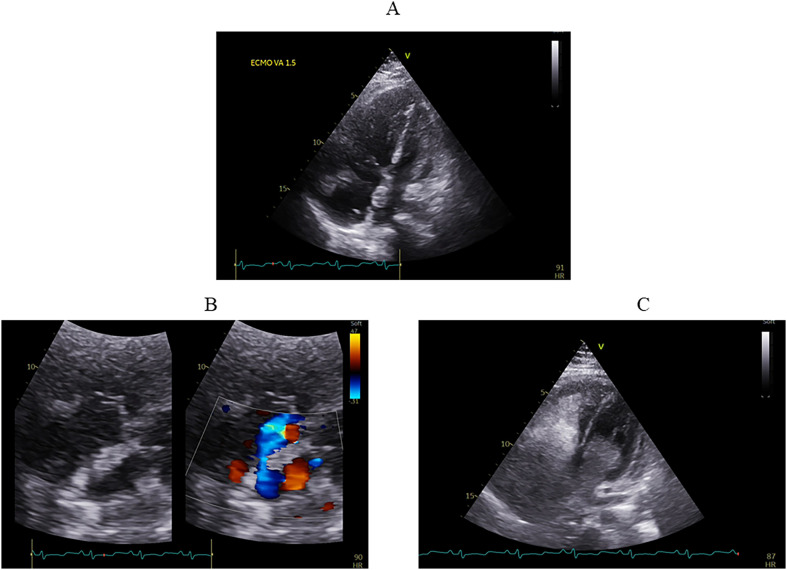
A. Apical four-chamber view on transthoracic echocardiography performed before atrioseptostomy (veno-arterial ECMO flow = 1.5 L/min). The view showed a dilated right ventricle, a compressed D-shape left ventricle, and a flattened septum. B. Transthoracic echocardiography with Color Doppler. A turbulent blood flow is visible through the interauricular septum. The flow appears bidirectional (with both blue and red signals mixed). The peak velocity is indicated around 47 cm/s, with a color scale ranging from −31 to +47 cm/s. C. Transthoracic echocardiography with a bubble contrast study. The bubble contrast study shows an opacification of the right-sided chambers and an immediate left heart opacification, suggesting an overt shunt.
